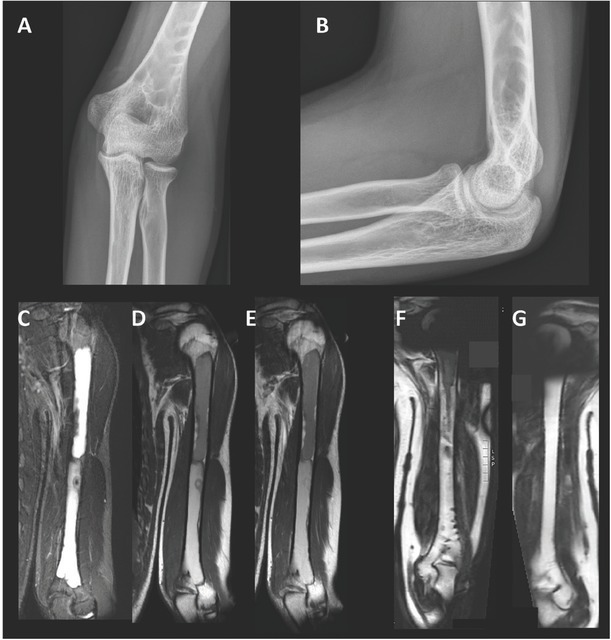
(A, B) Anterior–posterior and lateral X-ray of the left elbow at 17 years of age. Multiple lytic lesions are found in the medullary location of the distal humerus with thick sclerotic septae towards the cortex and a well-defined lower border (best appreciated on the anterior–posterior incidence). There is no significant cortical thinning or cortical scalloping nor periosteal reaction. Periarticular soft tissue and fat pads appear normal, no signs of hydrops; (C, D, E) nuclear magnetic resonance imaging (MRI) of the left humerus (17 years of age) shows abnormal heterogeneous signal intensity of the medullary cavity of the humerus shaft, hyperintense compared to the intensity of the muscle on the short tau inversion recovery (STIR) and T1-weighted (T1W) sequences. The lesion extends from the proximal to the distal end, is non-enhancing and contains septations as well as a non-specific slightly enhanced nodular lesion in the lower half of the humeral shaft. The difference in the intensities between the lower and upper halves is due to more haemorrhagic and protein-rich fluid in the upper half. These findings are compatible with a non-progressive large cystic lesion in the left humerus shaft. If there is no genetic confirmation of polyostotic fibrous dysplasia, one could consider the possibility of a lymphangioma; (F,G) on follow-up MRI (at 25 years of age), the lesion has decreased in size with a residual component in the proximal humerus and bony ridges in the distal humerus.
